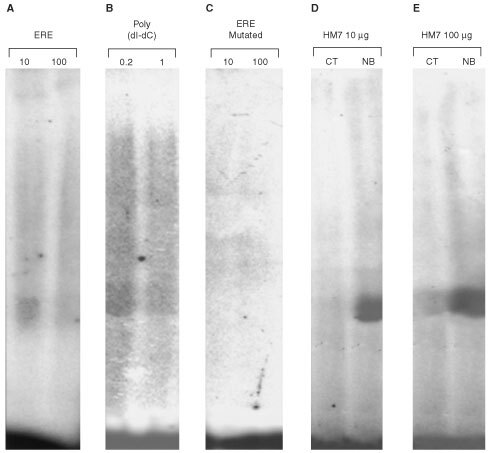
Butyrate-induced protein-ERE complex formation in a gel shift assay. DNA-protein complex formation was measured using ^32^P-labelled 22 bp ERE probe and nuclear extracts of HM7 cells. Competition in protein-DNA formation: 10 and 100 ng unlabelled ERE (A), 0.2 and 1 μg poly(dI-dC) (B) were incubated with HM7 nuclear extracts before adding labelled ERE probe. Binding specificity determined with 10 and 100 ng ^32^P-labelled C–A replaced ERE probe at the sites indicated by underline (5′-CCCTCTATCCAGCTCCTATCCC-3′) and incubated with nuclear extracts of HM7 cells (C, ERE mutated). Nuclear extracts from control (CT) or butyrate-treated (NB) cells were incubated with the labelled ERE probe. The binding was dependent on the concentration of the nuclear protein extracts (D; 10 μg, E; 100 μg). Representative results from three similar experiments.
